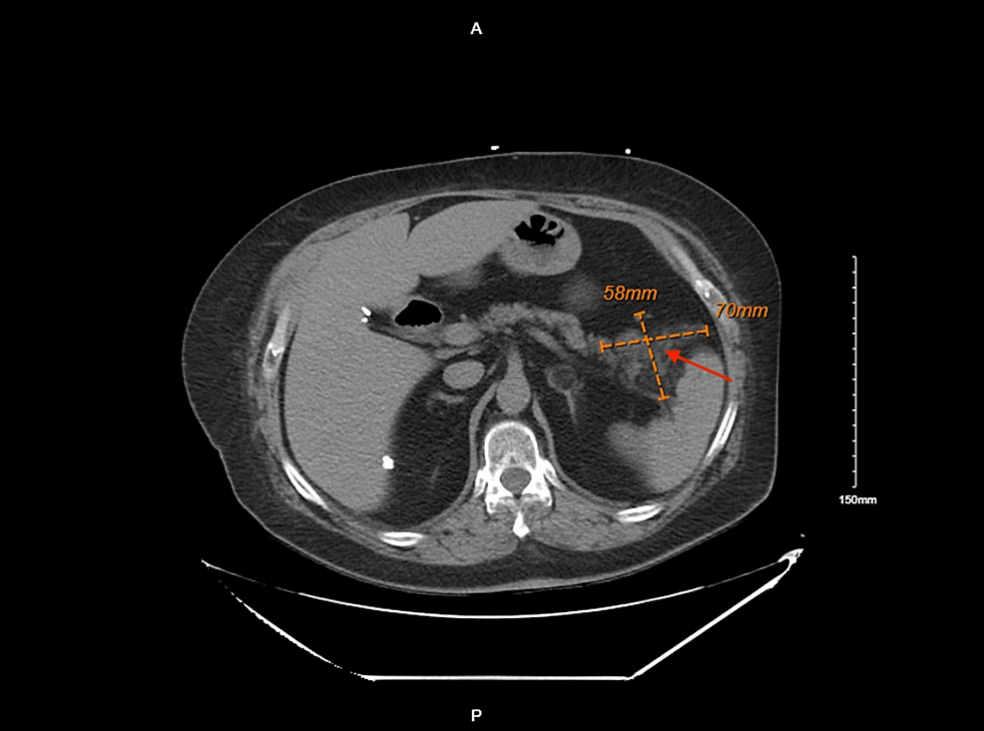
Computed tomography significant for a focal fluid collection (red arrow) seen at the tail of the pancreas measuring up to 7.0 cm x 5.8 cm or 70 mm x 58 mm in conglomerate cm: centimeters; mm: millimeters
